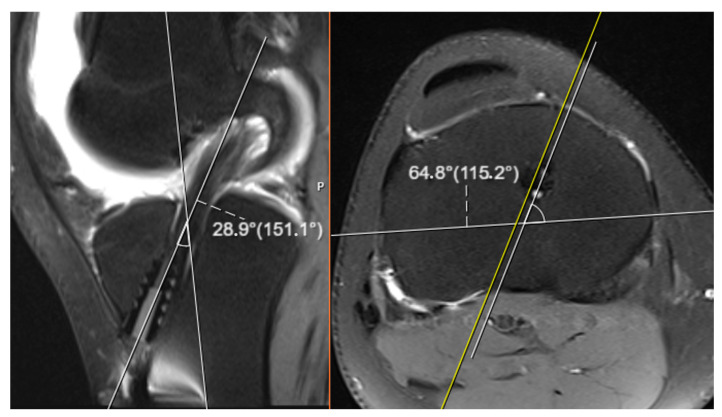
Image showing the measurement of tunnel angles on MRI.
